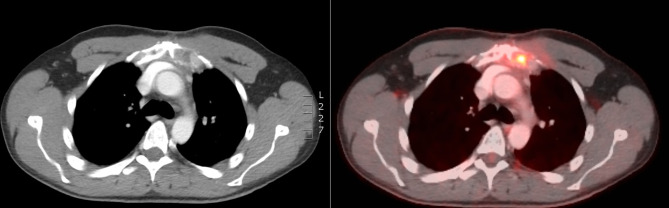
CT scan 7 months after the end of RT showing volume decrease of the sternal lesion.
